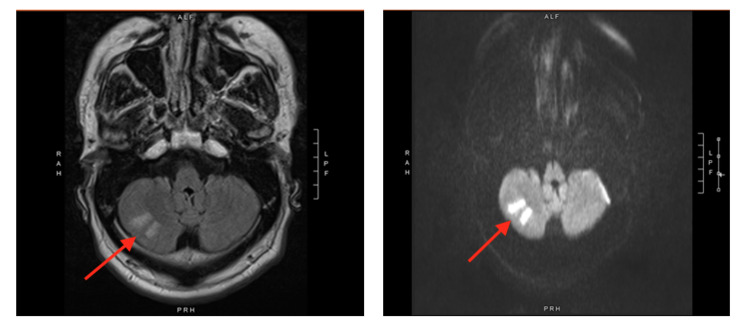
Magnetic Resonance Imaging of the Brain Red arrows depict acute/early subacute infarcts in the inferior aspect of the right cerebellum hemisphere.
